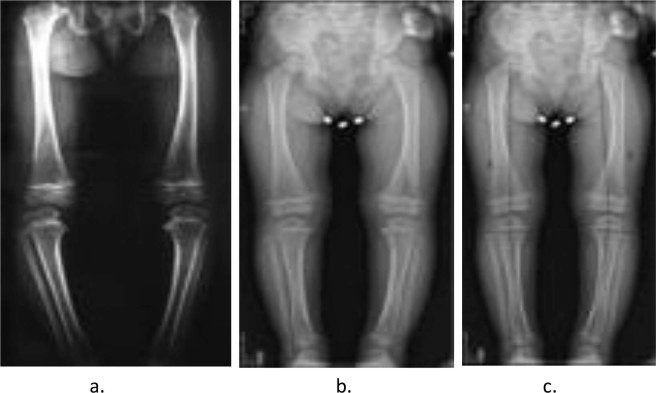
Anteroposterior radiographs of lower extremity, (a). Before treatment, (b,c). After treatment.
